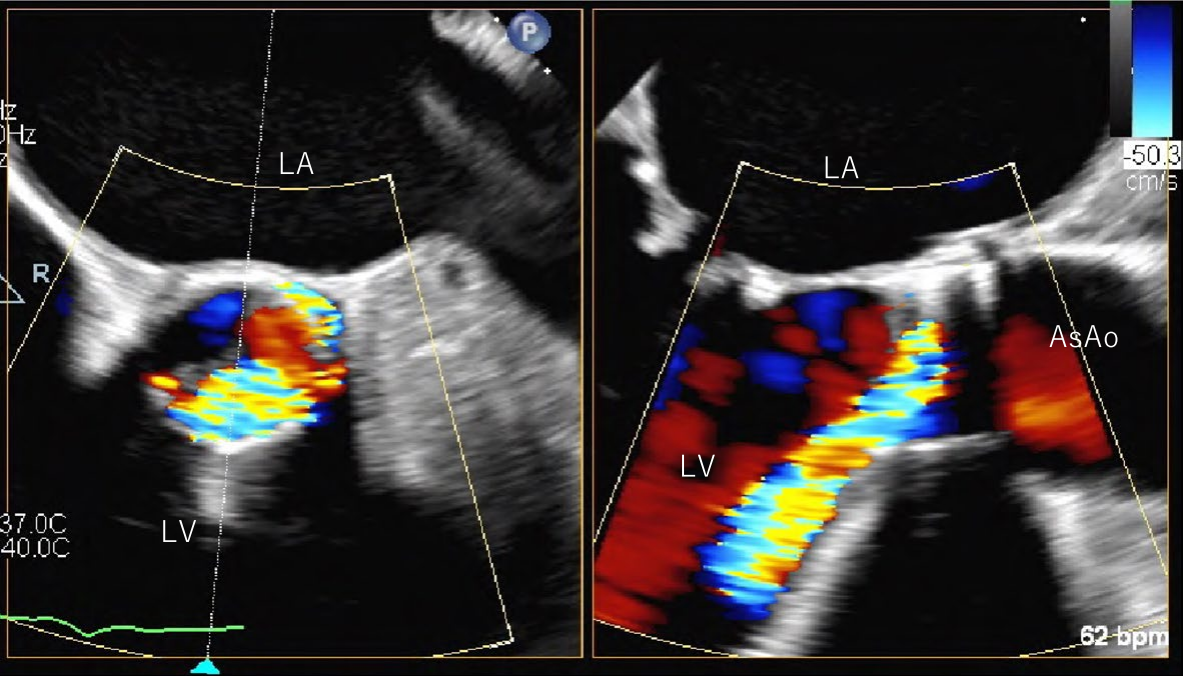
Preoperative transesophageal echocardiographic view of case 1
